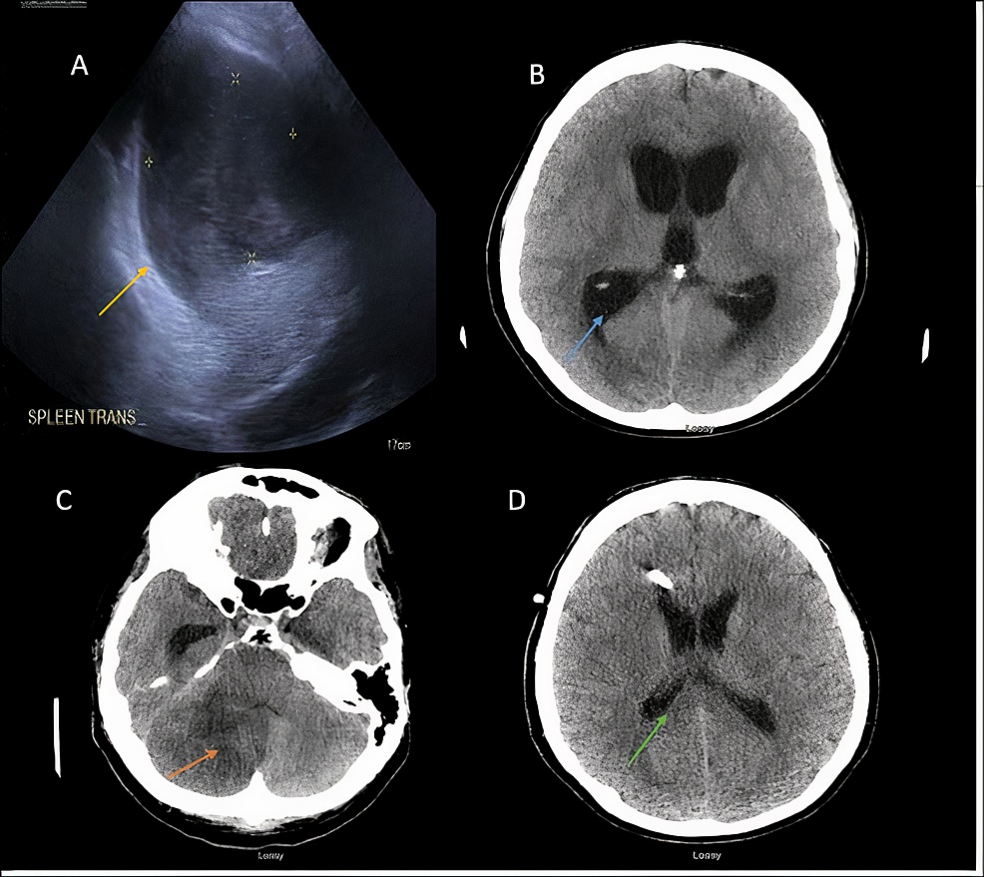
Ultrasound of the abdomen: large splenic abscess (yellow arrow in A). CT of the head without contrast showing acute hydrocephalus (blue arrow in B), and a large cerebellar infarct (orange arrow in C). Repeat CT of the head showing improvement in hydrocephalous (green arrow in D). CT: computed tomography
